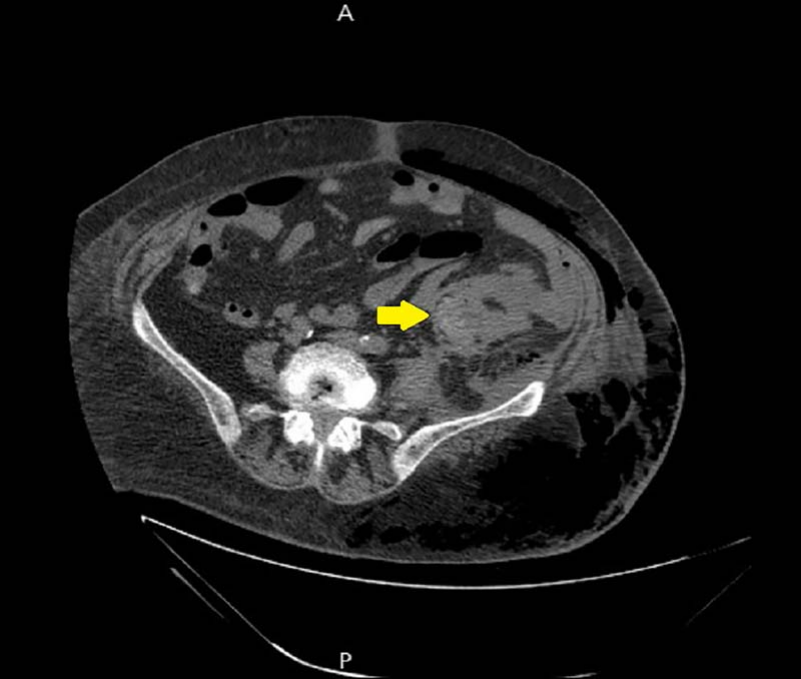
A non-contrast abdomen and pelvis CT scan, which shows a soft tissue mass lesion at the distal descending colon.
